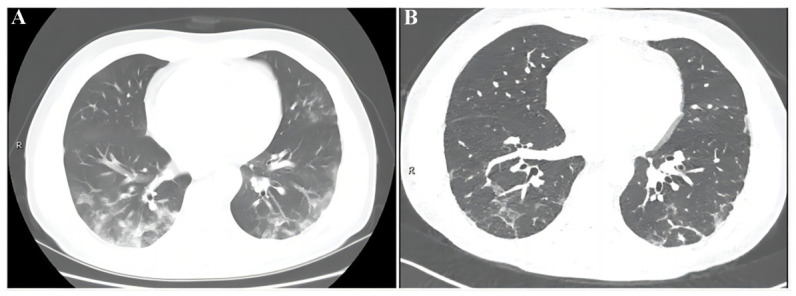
Comparison of CT imaging of the lungs before and after treatment: (A) pre-treatment shows multiple ground-glass opacities and consolidations (white areas) in the subpleural regions of the lower lobes; (B) post-treatment demonstrates marked reduction of these white areas.
